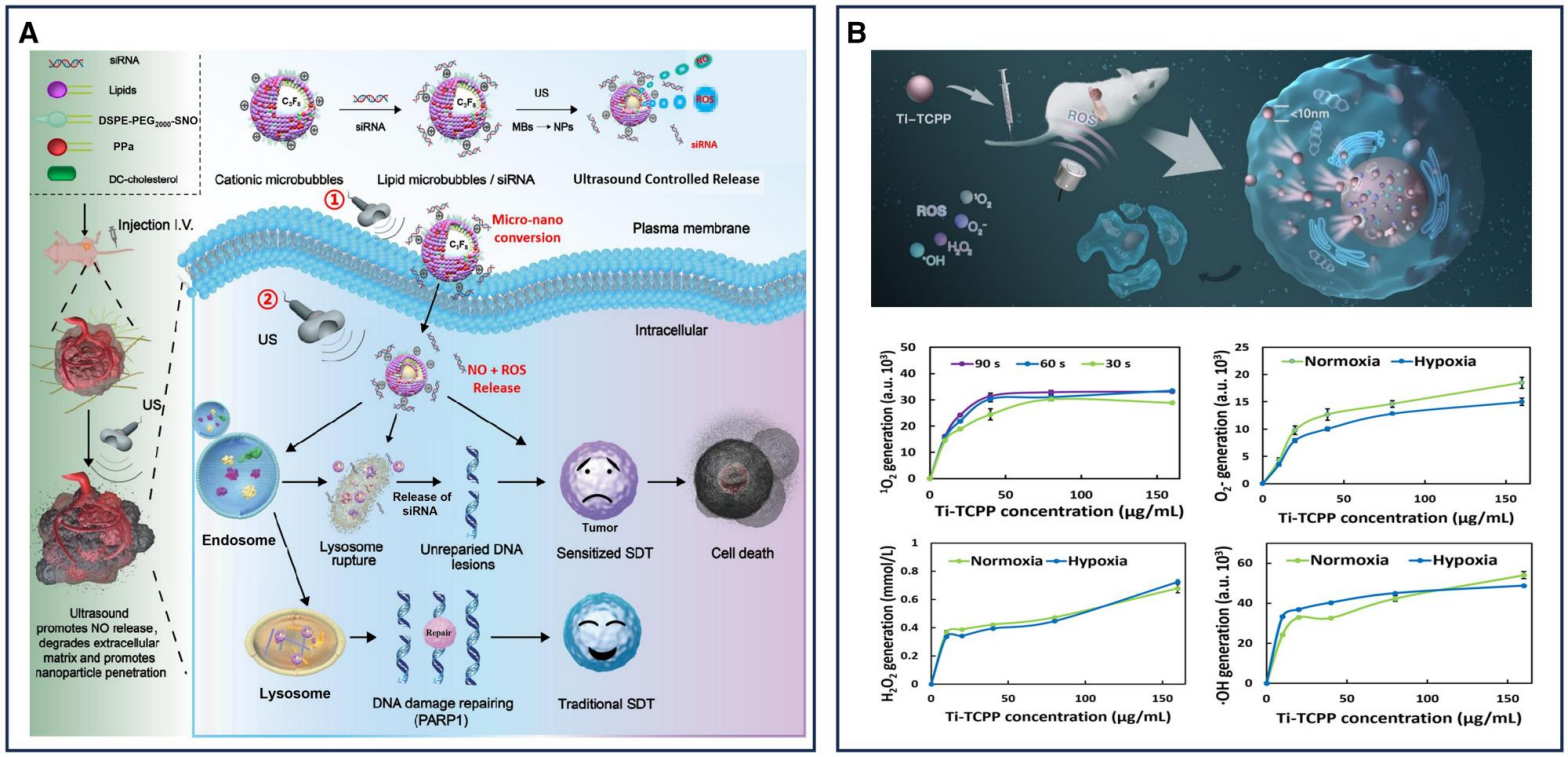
Nanomaterial-assisted sonodynamic therapy of pancreatic cancer. (A) Preparation of ultrasound-responsive functional microbubbles with membrane shells and adsorption of PARP1 siRNA on the surface for effective SDT of pancreatic cancer. Reproduced with permission [178]. Copyright 2024, Elsevier. (B) Ultra-small Ti-TCPP MOF facilitates the delivery of acoustic sensitizers and increases ROS production. Reproduced with permission [179]. Copyright 2021, Springer Nature.
